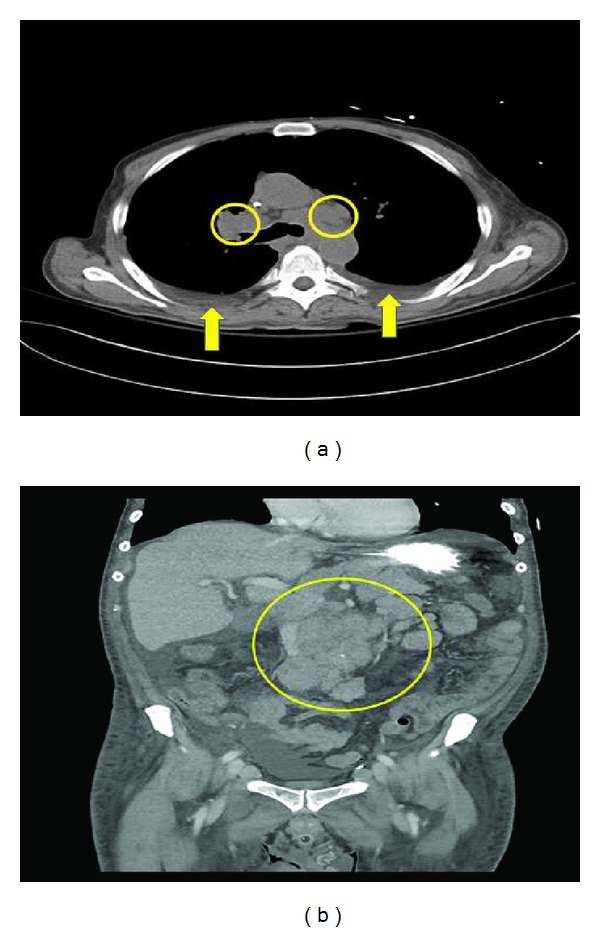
(a) CT imaging of the chest demonstrating bilateral pleural effusions (arrows) and mediastinal adenopathy (circles) and (b) CT imaging of the abdomen demonstrating bulky mesenteric adenopathy (circle).
